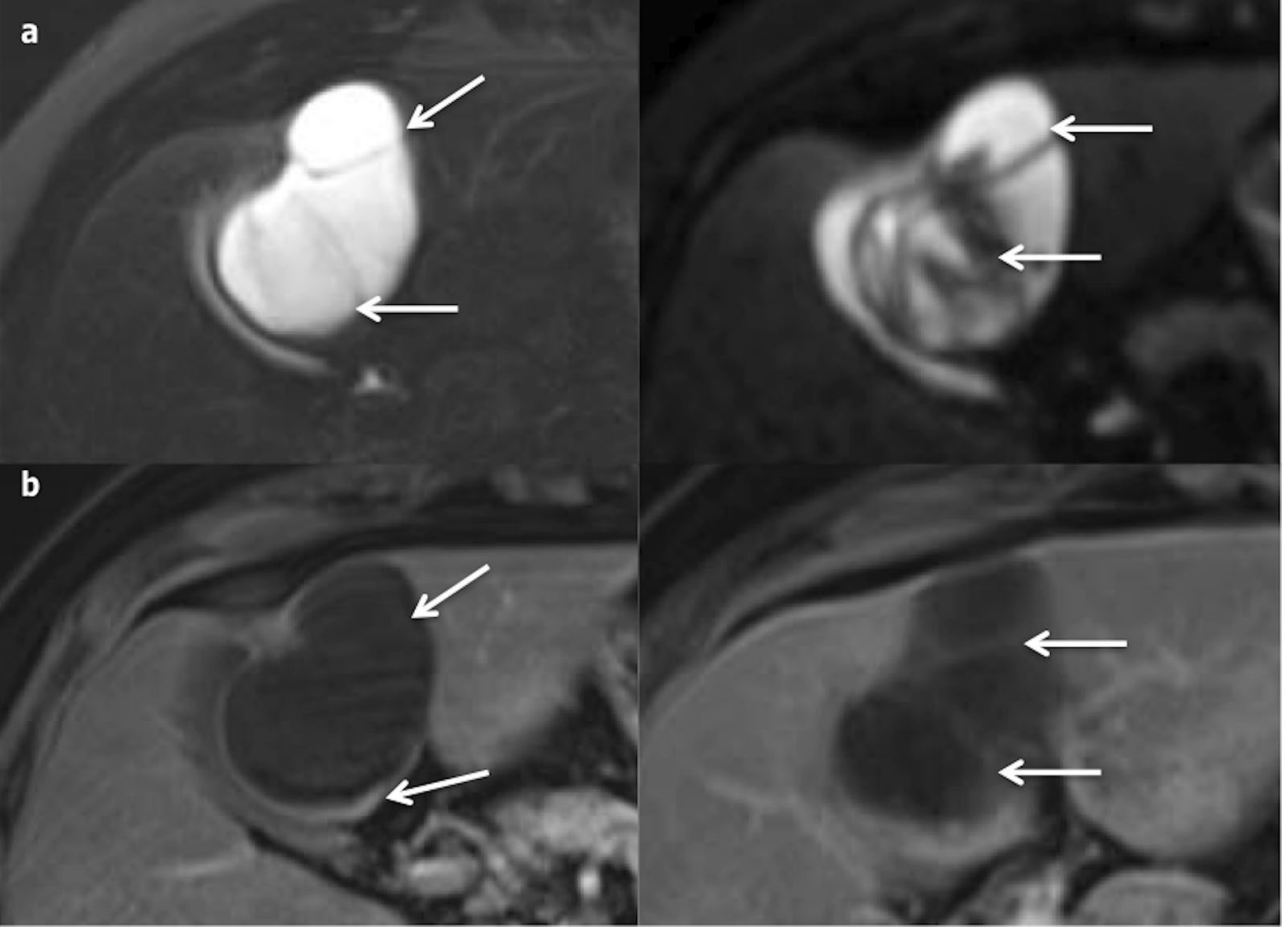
Typical non-invasive mucinous cystic neoplasm of the liver in a 59-year-old female. a Axial T2-weighted magnetic resonance imaging shows a solitary hyperintense cystic lesion, with hypointense septa (arrows). b Axial T1-weighted magnetic resonance imaging on portal venous phase depicts the enhancement of the capsule and septa (arrows)
